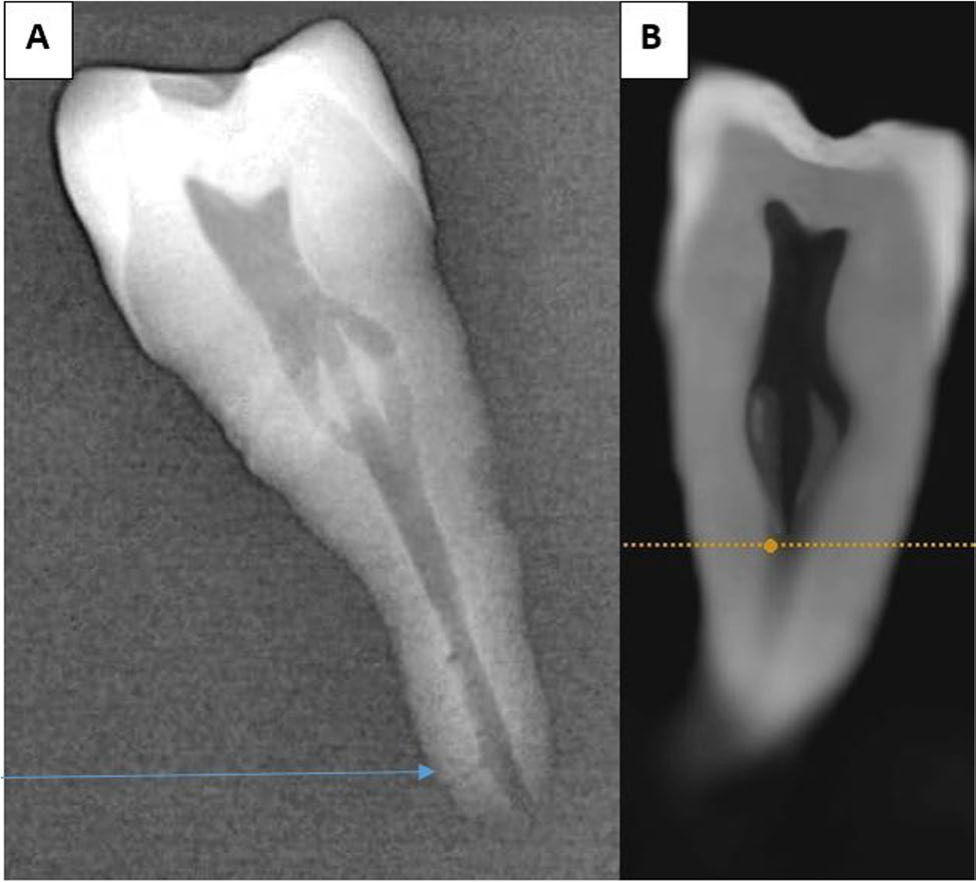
The additional classification observed in a second MP identified as 3-1-2 according to Vertucci’s classification and ^1^TN ^3−1−2^ according to Ahmed’s classification. A: captured using digital sensor imaging B: one of the CBCT slices. The arrow highlights the small additional canal, which altered the classification and was detected in the digital sensor image but was not identified in the CBCT sections
